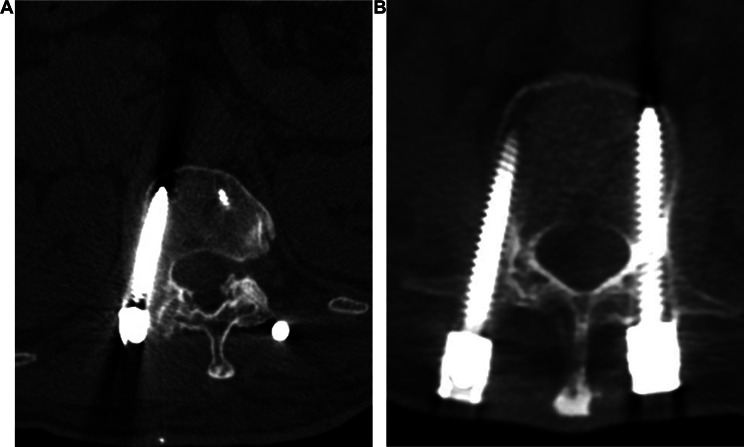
Postoperative computed tomography (A-B) revealed that the screws were positioned outside the lateral wall of the pedicle
